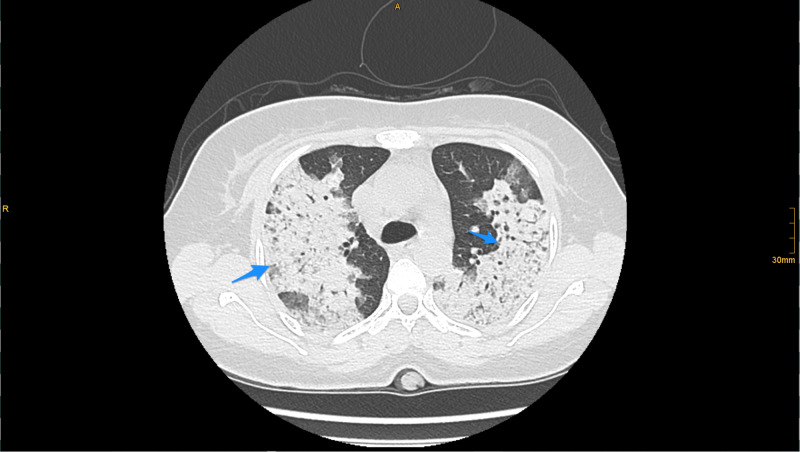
CT chest (lung window) CT chest (lung window) demonstrated a widespread patchy consolidation with central and peripheral distribution, permeated by ground glass areas and crazy paving pattern. These findings are suggestive of diffuse alveolar hemorrhage (blue arrows).
